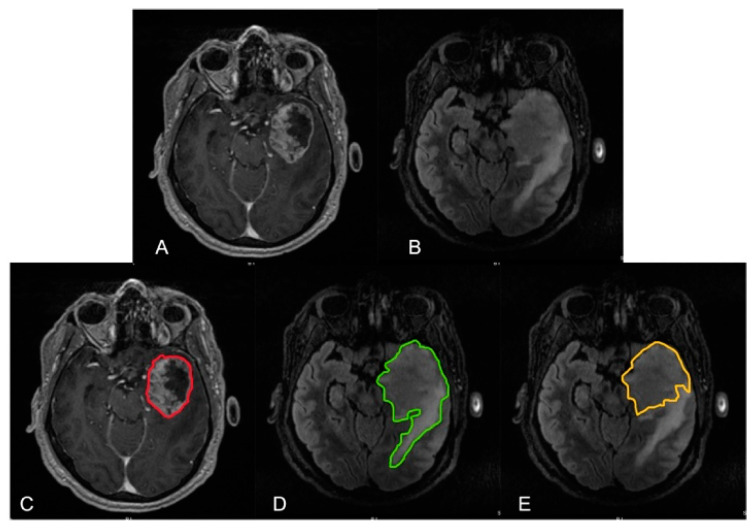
Preoperative magnetic resonance imaging highlighting left temporal lobe glioblastoma. (A) T1 post-contrast MRI. (B) T2 FLAIR MRI. (C) Red line highlighting contrast-enhancing tumor. (D) Green line demonstrating extent of tumor associated FLAIR. (E) Gold line outlining potential extent of non-contrast enhancing tumor or mass-like FLAIR signal.
